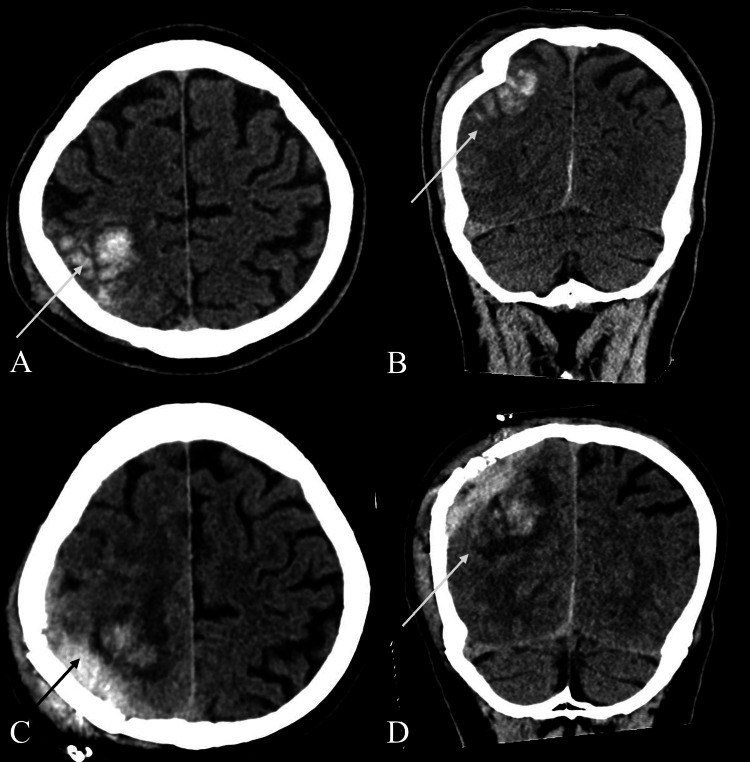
CT Brain Images for Patient 2 (A) Axial view demonstrating right intraparenchymal cortical/subcortical hematomas of the right parietal lobe; (B) Coronal view demonstrating depressed fracture of the right parietal bone, with intraparenchymal hematomas; (C) Axial and (D) Coronal views performed on POD 8, demonstrating increased edema in the right parietal lobe. CT, computed tomography; POD, post-operative day
